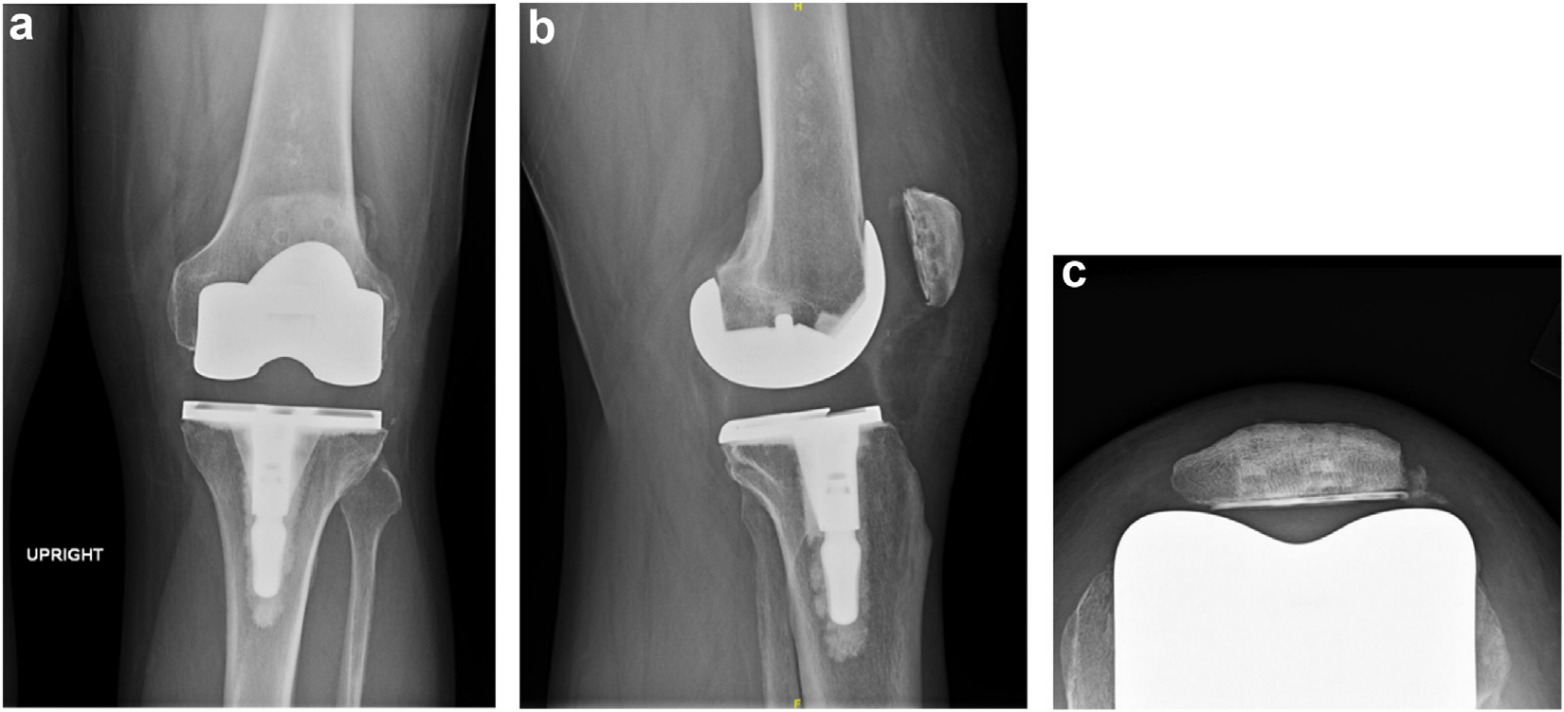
(a-c) Prefailure radiographs at 4 months postoperatively of primary TKA showing anteroposterior, lateral, and sunrise views. Note the radiolucency between the cement and the patellar bone, best observed on the sunrise view. In hindsight, this may have been an early indication of failure at the cement-bone interface.
